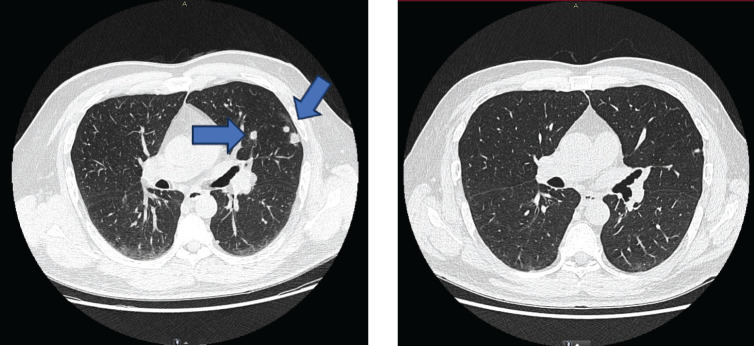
Evolution of the patient's computed tomography scans over different time periods. Left: CT scan at the beginning of immunomodulatory therapy (30 December 2016) – showing multiple solid pulmonary nodules (8–13 mm) predominantly in the peripheral left mid upper and lower lobes and left hilar adenopathies (15–17 mm). Right: CT scan (8 July 2022) – showing complete response of thoracic lesions after 50 cycles of immunomodulatory therapy.
